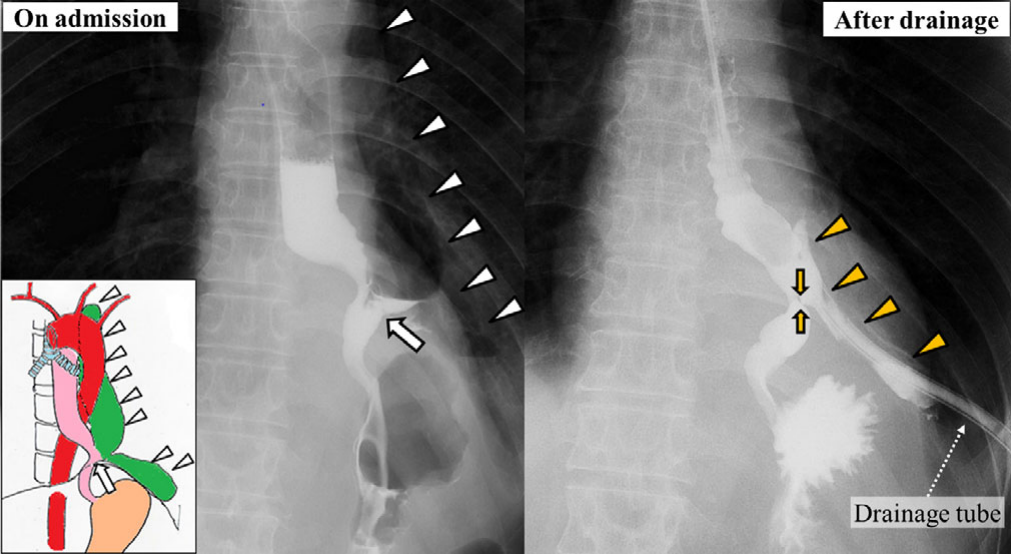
Gastrografin upper gastrointestinal contrast radiography on admission and after preoperative abscess drainage. On admission, a 2‐cm diameter fistula (white arrow) was detected in the left wall of the thoracic lower esophagus. The fistula was continuous with an abscess in the left thoracic cavity (white arrowheads). After preoperative abscess drainage, gastrografin radiography via the preoperatively inserted gastric tube and left abscess drain revealed that the fistula (orange arrows) and abscess (orange arrowheads) had both decreased in size
